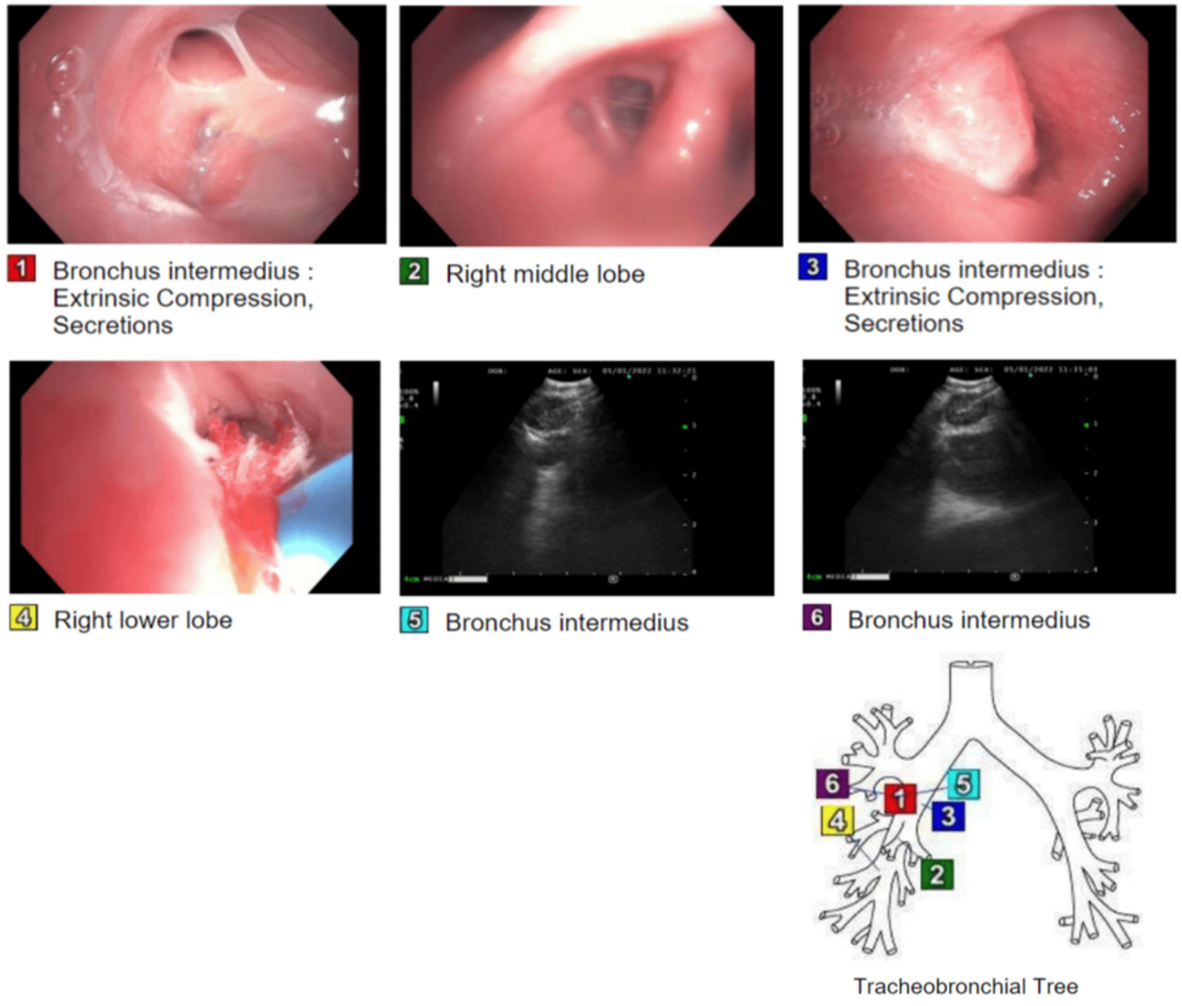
Bronchoscopy identifying a right hilar mass, extrinsic compression of the bronchus intermedius with the airway lumen nearly occluded secondary to posterior mass effect, and notable secretions throughout the right tracheobronchial tree. Transbronchial needle aspiration, lung biopsy, brushings, bronchoalveolar lavage, and endobronchial ultrasound performed. This image is the original work of the authors.
